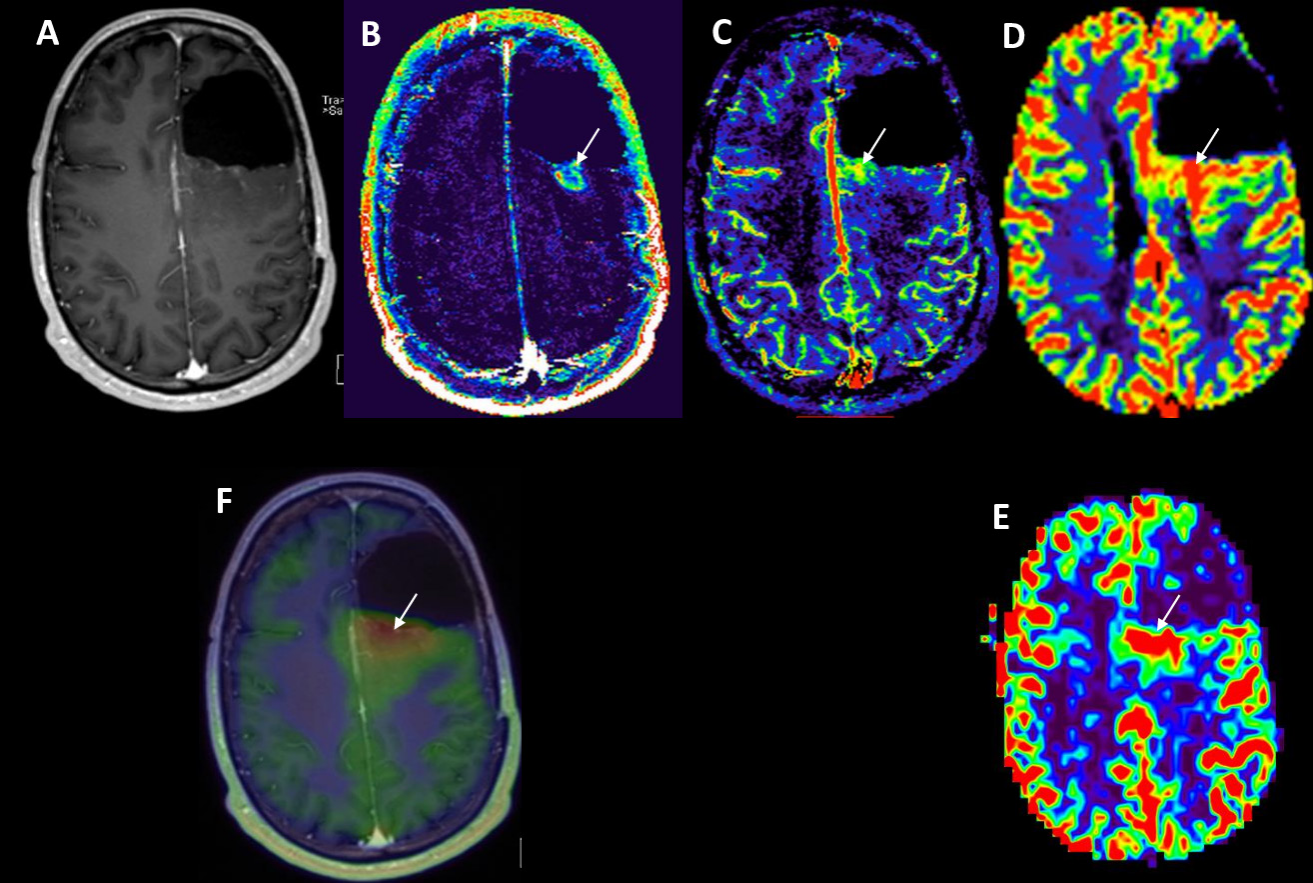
Multiparametric hybrid MR-PET imaging in a patient with IDH-mutant, WHO Grade 3 astrocytoma on the left frontal lobe treated with surgery and adjuvant chemoradiation. Progressive signal changes in the T2FLAIR images (not shown) with some tiny patchy enhancement (A) prompted the comprehensive MR-PET exam. Pathological changes related to neovascularity are visible in the permeability map (B) and intravascular blood volume (C) derived from the DCE with slight incongruence in their spatial distribution zone, reflecting the different pathophysiology that these biomarkers may capture. The intravascular blood volume map is more in line with the DSC-derived rCBV map (D) and the blood flow map from the arterial spin labelling measurement (E). (F) The fused post-contrast *T*_1_WI with the FET-PET image shows avid tracer uptake in the area of hypervascularity. FLAIR, fluid-attenuated inversion recovery; PET, positron emission tomography; *T*_1_WI *T*_1_ weighted imaging.
